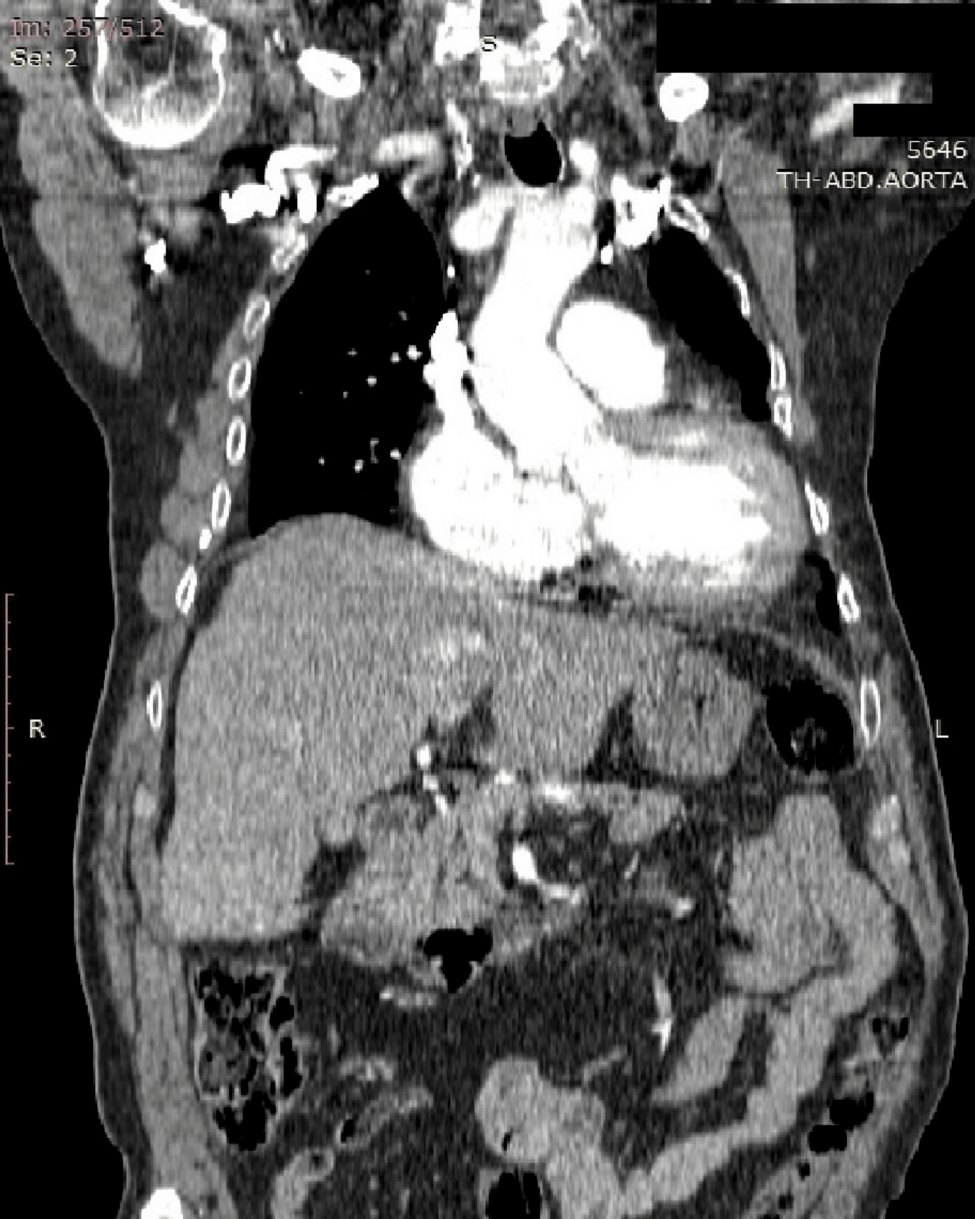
Preoperative coronal computed tomography view of the patient.
